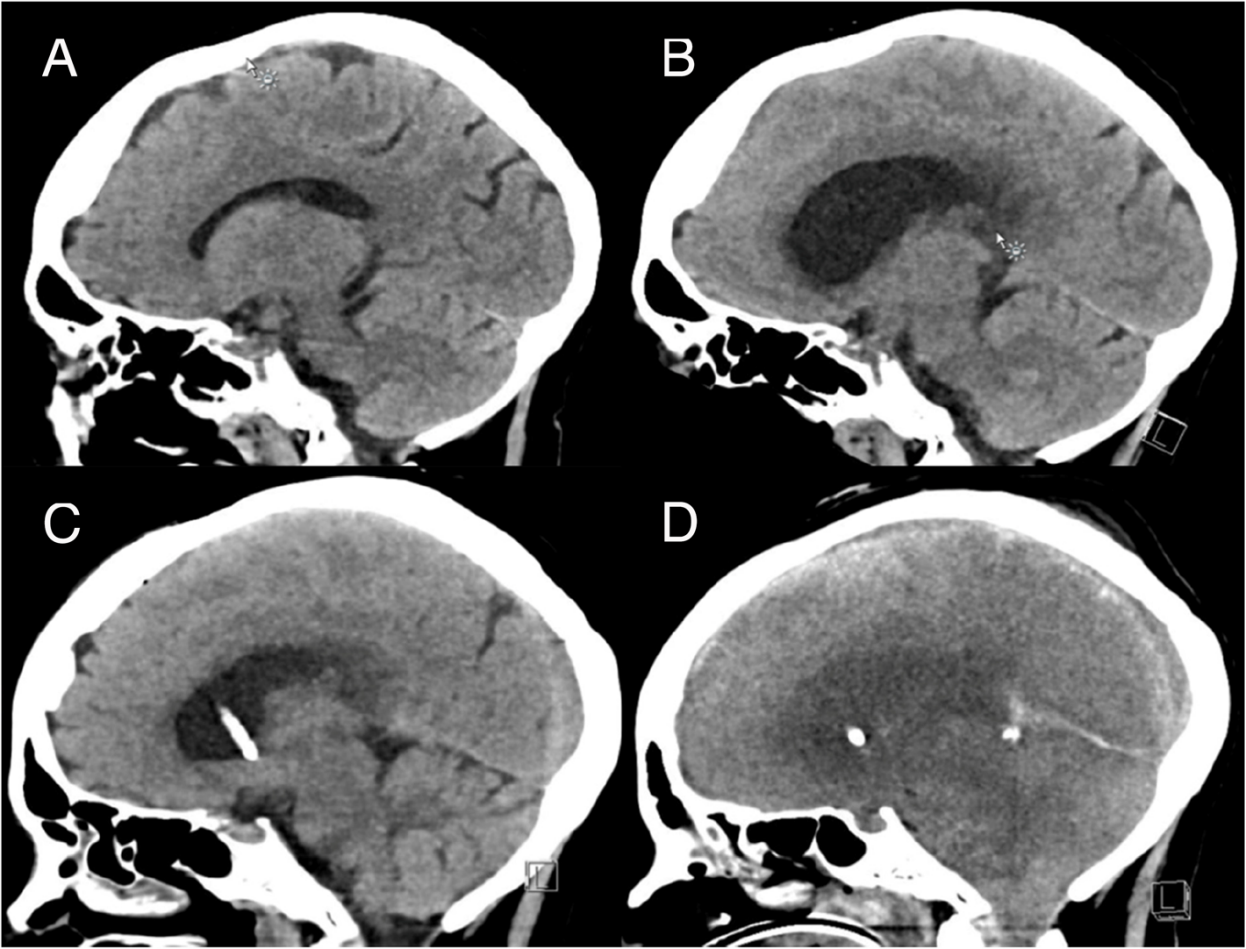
a Brain computed tomography at time of diagnosis, without particular complications. b Supratentorial hydrocephalus with loss of gyri. c Placement of ventriculostomies (bilateral, cannot be seen on the image) with reduction of the volume of the ventricular system. d Ventriculostomies *in situ*, although further development of edema and cerebellar herniation
